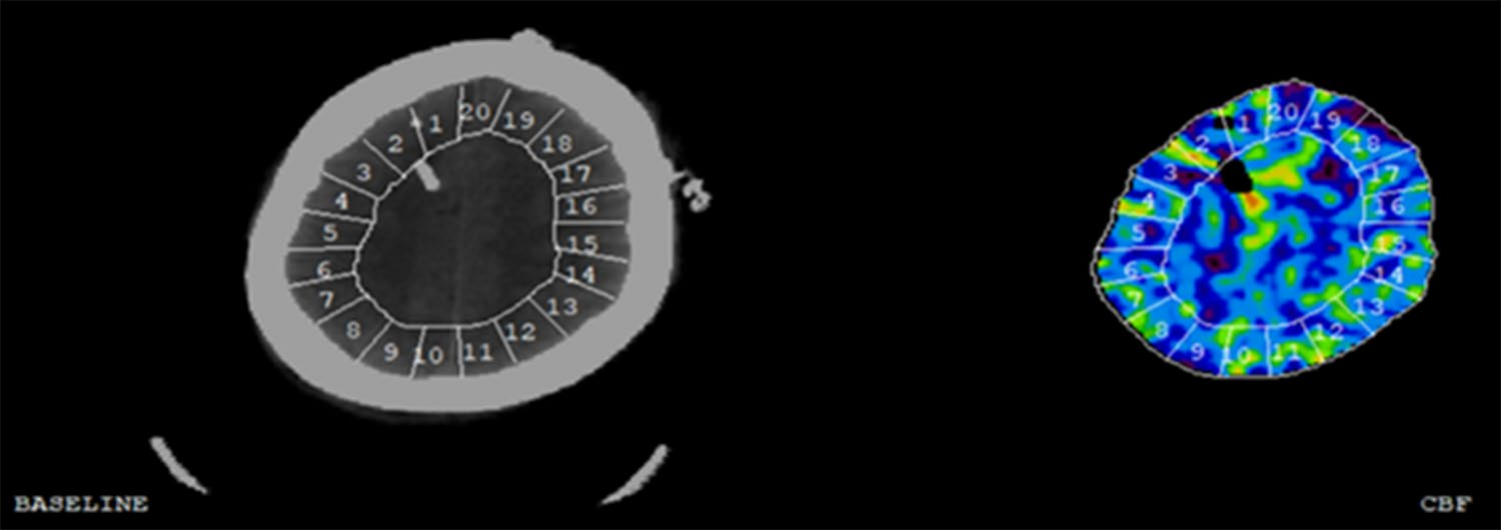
Cortical cerebral blood flow measurement—one example. The figure demonstrates cortical CBF in 20 different ROIs in a Xe-CT image. Global CBF was calculated based on average cortical CBF of typically three Xe-CT slices from the scan. Red areas indicate high CBF and blue areas low CBF. *CBF* cerebral blood flow, *ROI* region of interest, *Xe-CT* xenon-enhanced computed tomography
